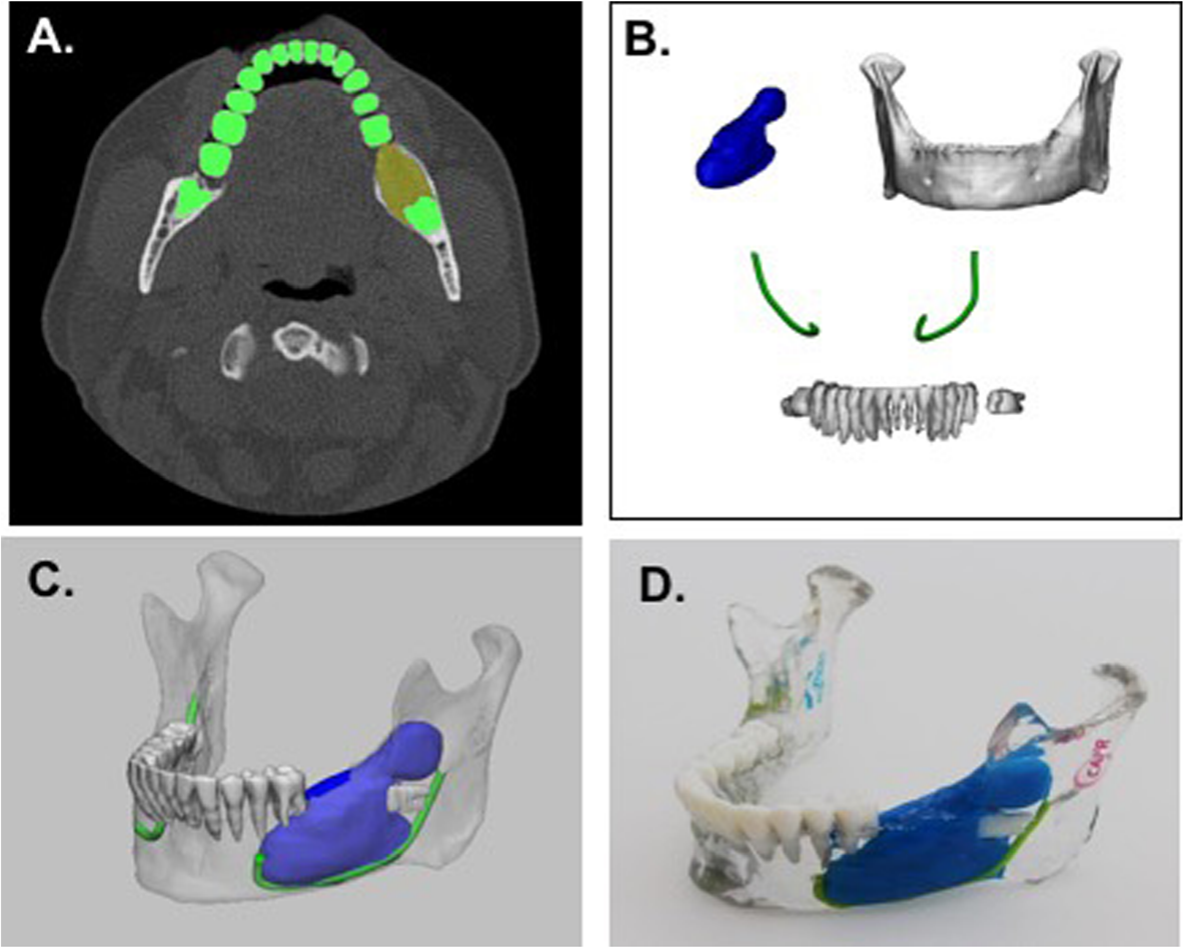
a Axial CT image showing segmentation of teeth (green) and tumor (yellow). b 3D anatomical regions of interest including the tumor (blue), mandible (white), teeth (white), and nerves (green). c 3D visualization of model including all anatomical parts. d 3D printed mandible tumor model including the mandible (clear), teeth (white), tumor (blue), and nerves (green)
